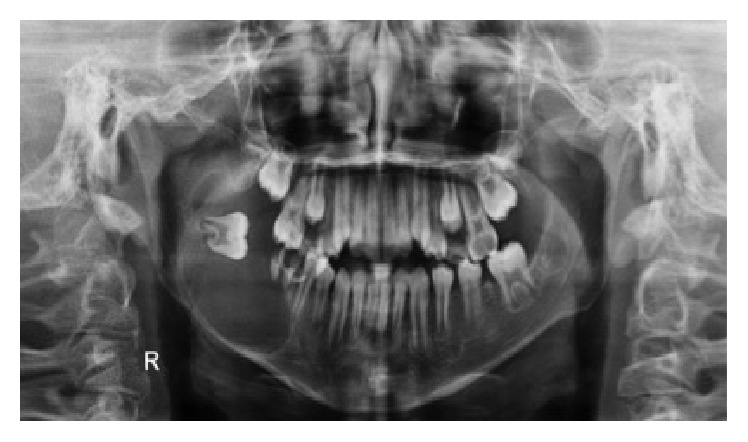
Panoramic radiograph: radiolucid image of right mandibular body and ramus, with involvement of the included tooth #47.
